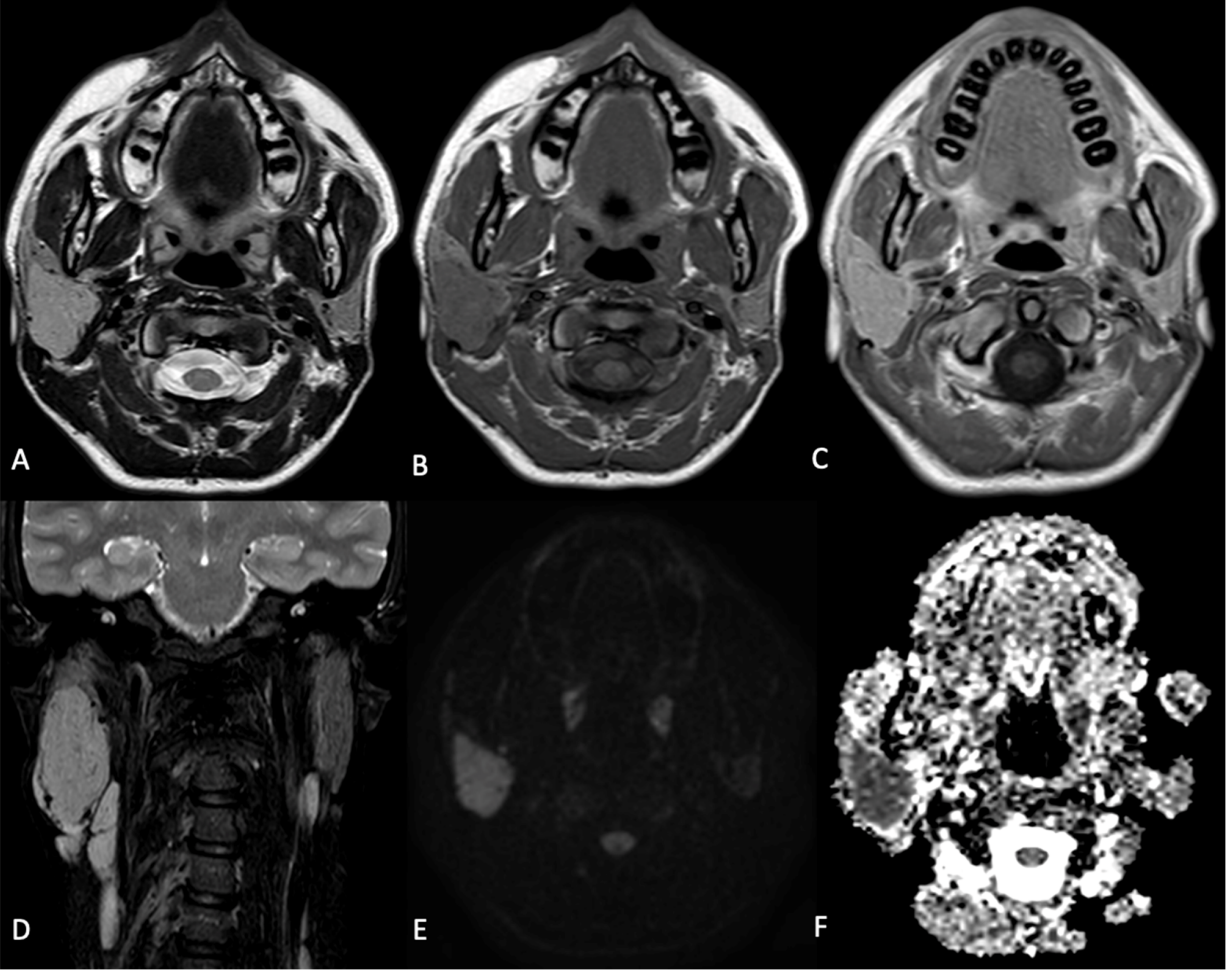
Axial T2WI (A), T2WI (B) and T1WI after contrast medium (C) demonstrate a well-defined solid lesion within the right parotid gland, which is hypo-intense on T1WI, relatively hyperintense in the T2WI image and hyper-intense on T1WI with contrast. There is only a mild diffusion restriction on DWI (E) and ADC maps (F). On coronal suppressed T2WI (D), and on axial T2WI (A), we observe some flow-voids images, with expression of high-flow blood vessels, confirmed on T1WI after contrast medium (C). Technique: 1.5T MR scanner. 3.0 mm slice thickness.
